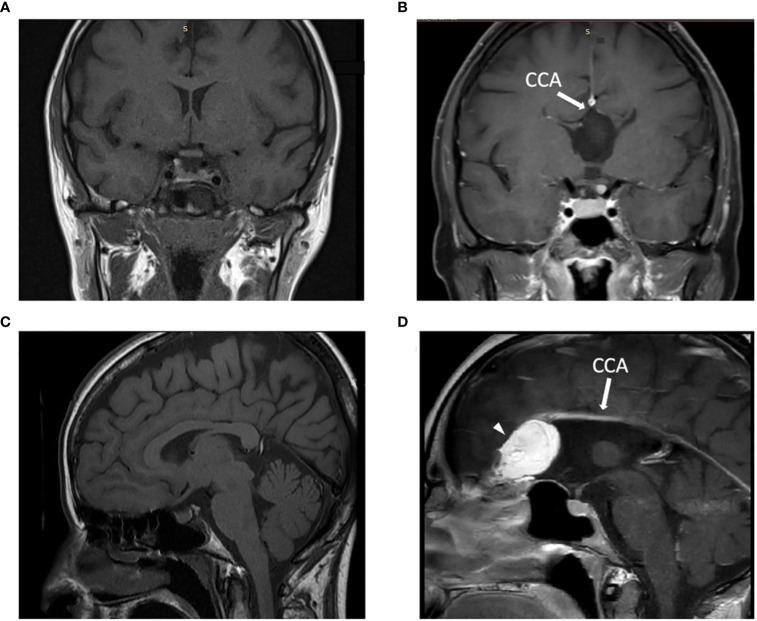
Patient’s MRI imaging and comparison with a control subject: T1-weighted MRI image in the coronal (A, B) and in the sagittal (C, D) plane demonstrated the presence of the corpus callosum (A-C) in a control subject and the corpus callosum agenesis (B-D) in our patient, indicated with white arrow. In D an arrowhead indicates a hyperintense lesion on the left frontal lobe consistent with intracranial teratoma. CCA: corpus callosum agenesis. Of note, the control subject is a female patient that performed MRI as follow-up radiological evaluation in the context of a clinical history of non-functioning pituitary adenoma, which underwent successful transsphenoidal neurosurgery.
